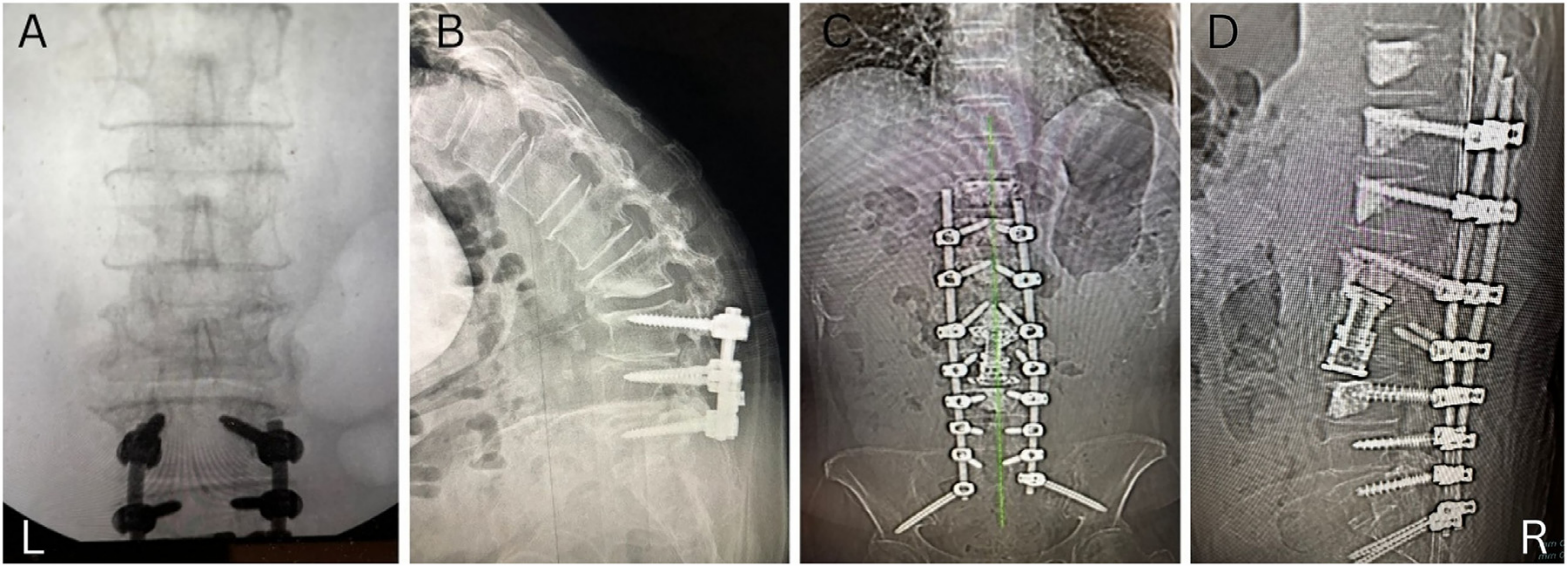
A. Preoperative anteroposterior radiograph demonstrating kyphosis above the fusion. B. Preoperative lateral radiograph demonstrating kyphosis above the fusion. C. postoperative anteroposterior radiograph demonstrating kyphosis correction. D. Postoperative lateral radiograph demonstrating kyphosis correction.
